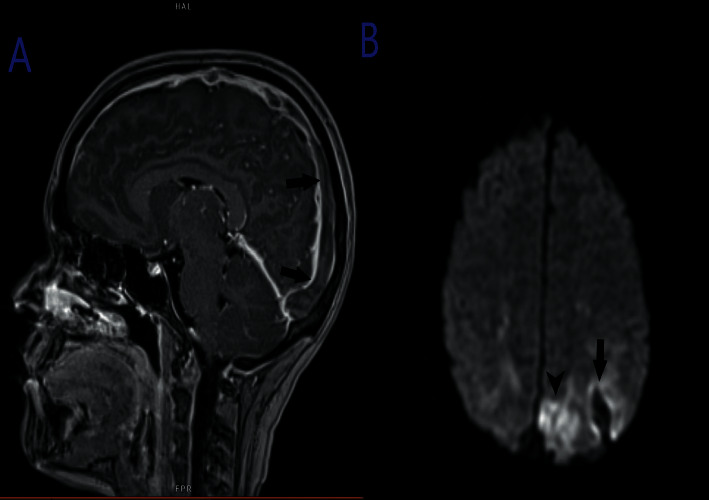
Magnetic resonance imaging (MRI) of the brain with contrast. (a) Postcontrast sagittal view showing extensive filling defect in the superior sagittal sinus consistent with thrombosis. (b) Diffusion weighted imaging (DWI) coronal view demonstrating left parietal lobe infarct (arrow) and hemorrhage (arrowhead).
